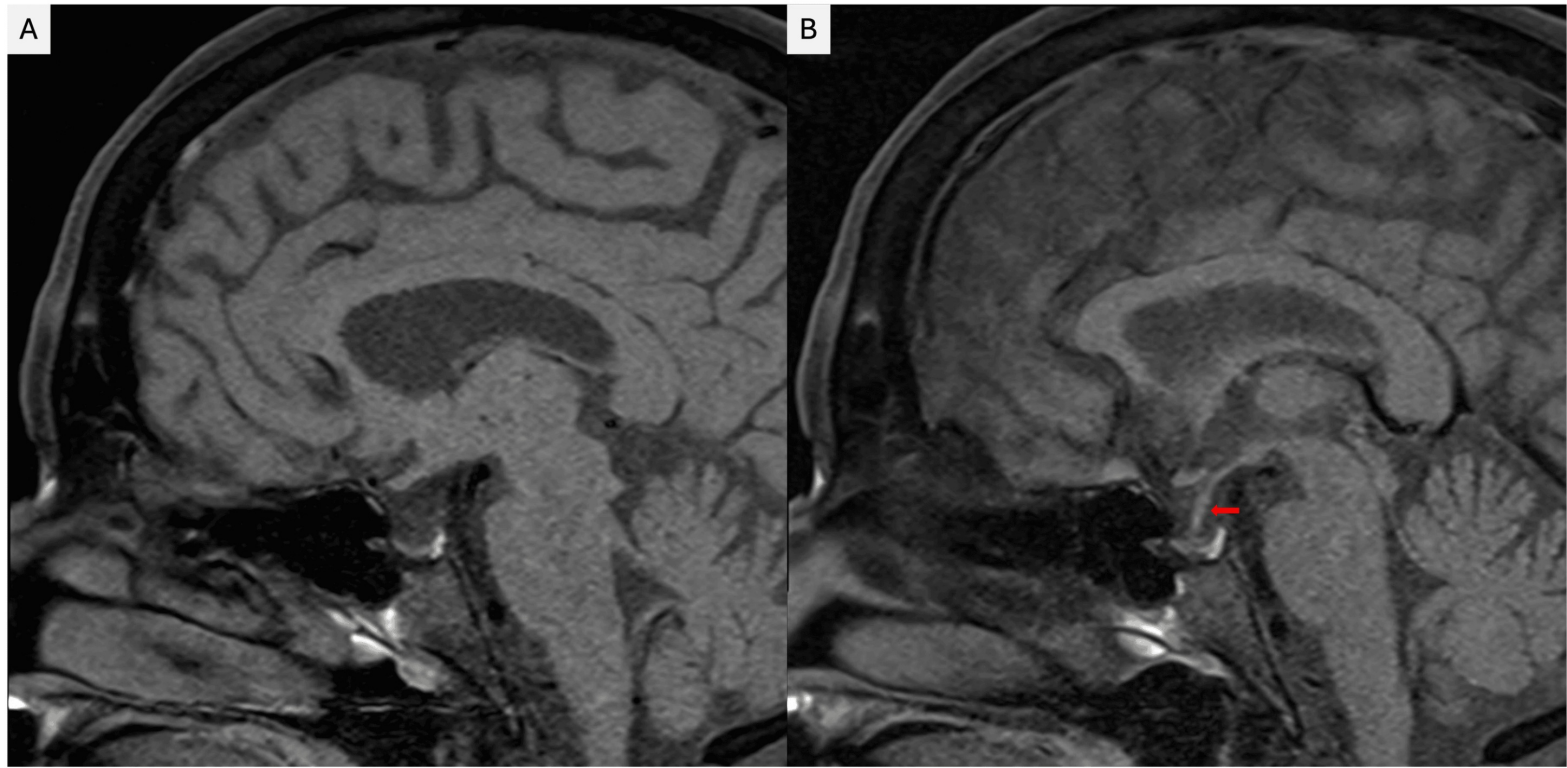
MRI of the mid-sagittal section of the brain showing (A) partially empty sella turcica; (B) Pituitary stalk (infundibulum) extends downward to the sellar floor (Red arrow).
